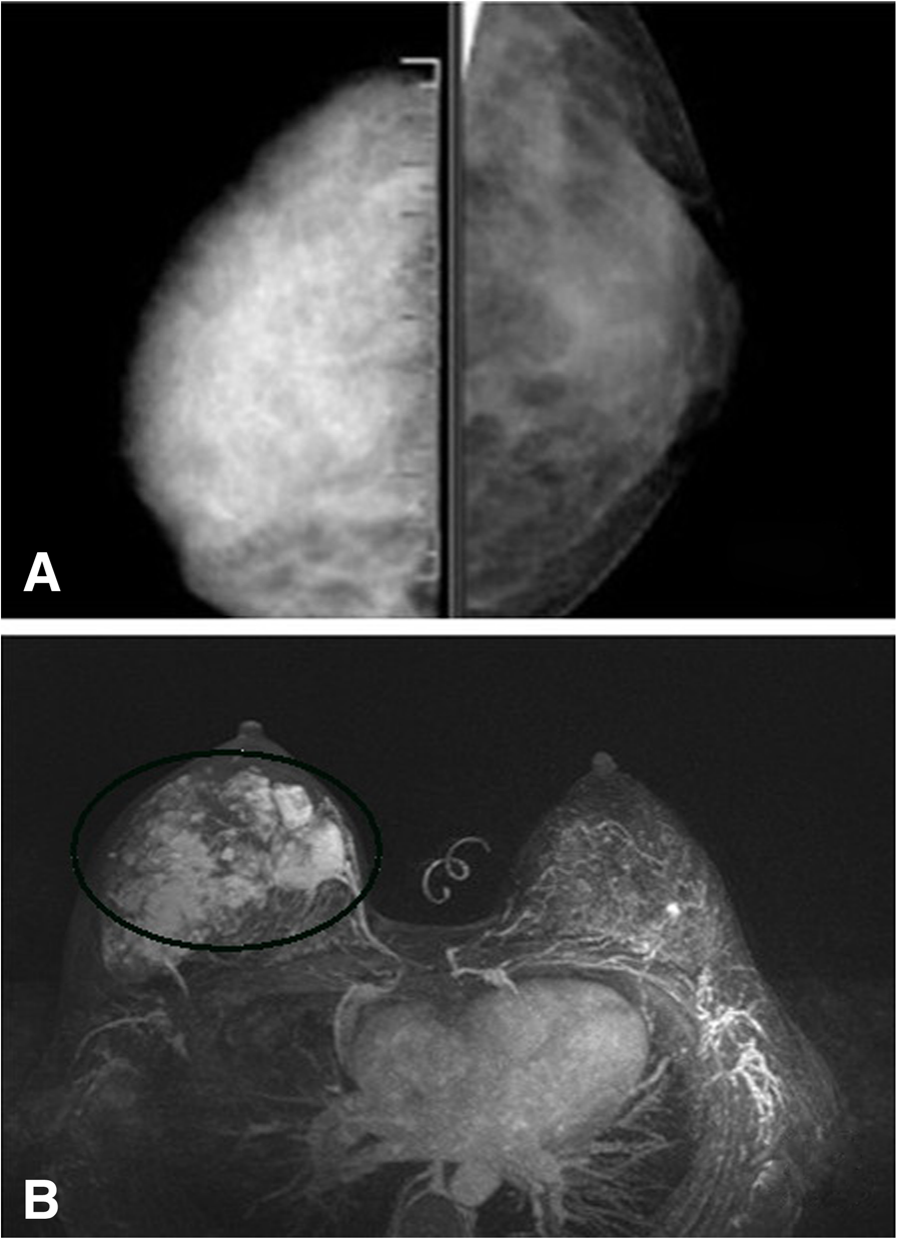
A female whose right breast has been swollen for 11 months. a: Diffuse dense shadow is observed in the craniocaudal view of the right breast. b: The contrast enhanced MR images reveal multiple heterogeneous enhanced masses
